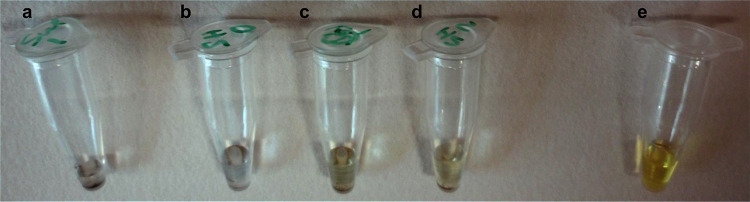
Ellman’s assay for the presence of free sulphhydryl (thiol) groups applied to edge-modified GNPs produced by ultrasonic exfoliation: a negative control containing 30 µl of graphene sulphonate and 10 µl Ellman’s reagent solution; b negative control containing 30 µl thiographene and 0 µl Ellman’s reagent solution; c 30 µl thiographene and 5 µl Ellman’s reagent solution; d 30 µl thiographene and 10 µl Ellman’s reagent solution; e positive control containing 30 µl 260 mM cysteine and 10 µl Ellman’s reagent solution. [Ellman’s reagent] = 10 mM; [graphene suspensions] = 0.5 g l^−1^
